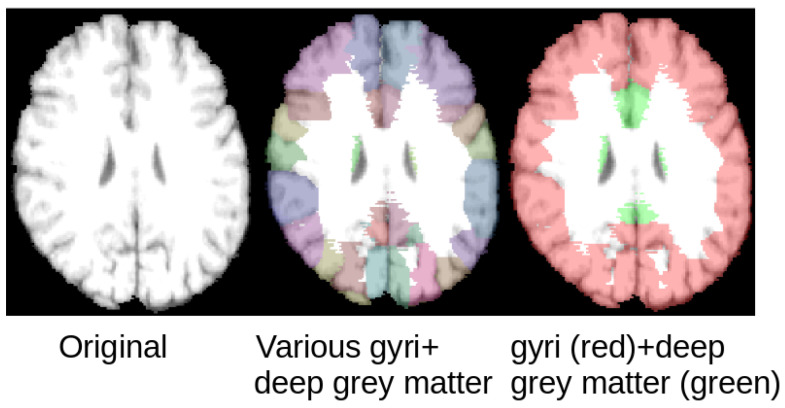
Selected slice from a MRI dataset from the LPBA40 database (left) with expert labels superimposed on the original slice (middle) and the fused labels used in this work (right).
